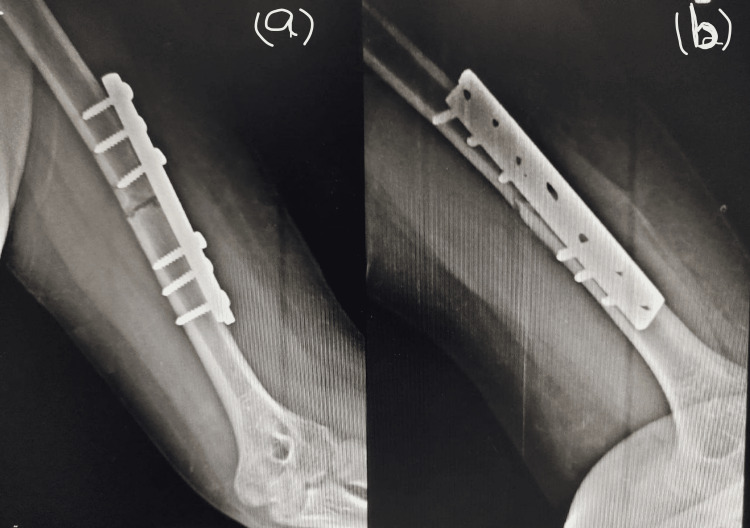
Radiograph showing the fracture site 11 weeks after the internal fixation Radiograph of left humerus (a) anteroposterior view, (b) lateral view
